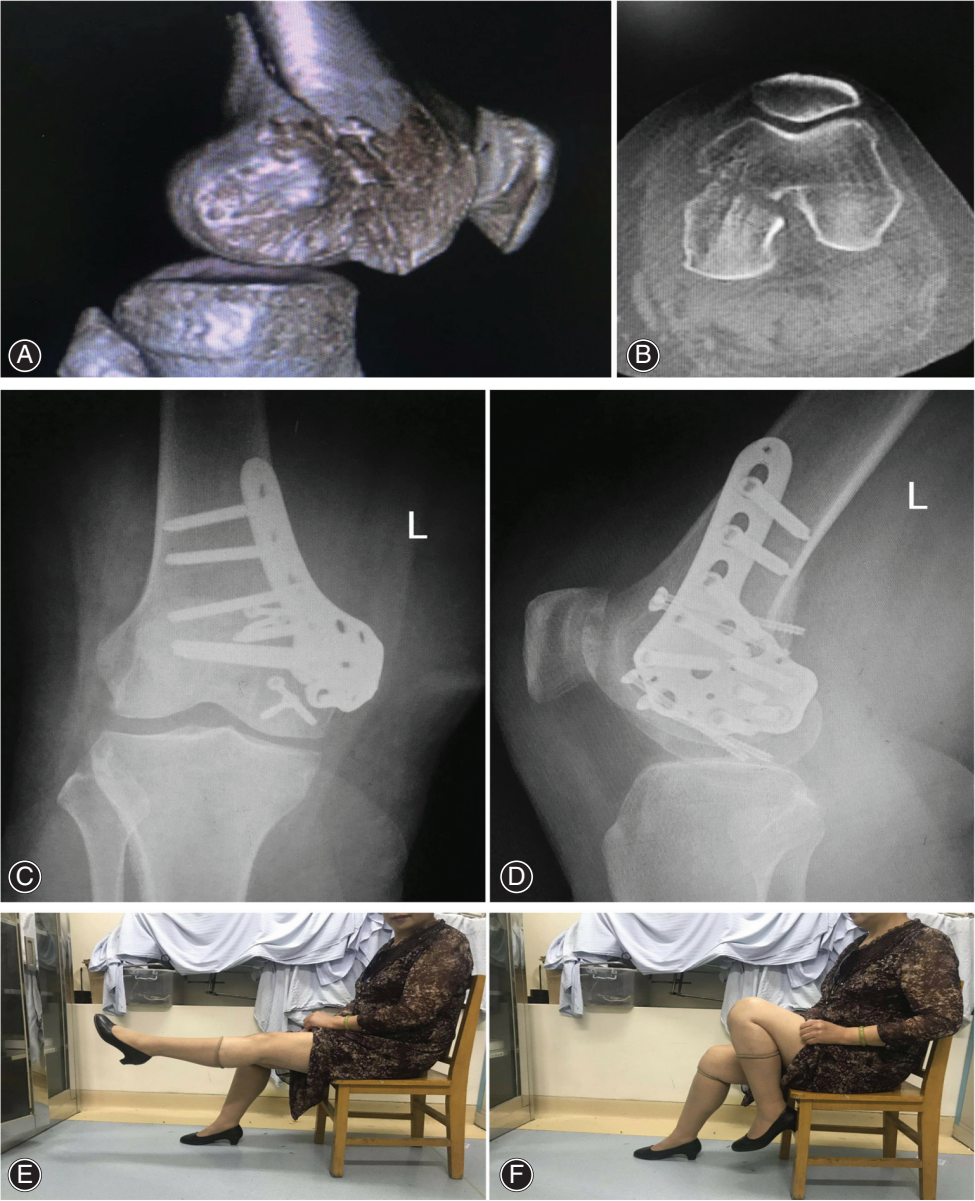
A 60‐year‐old female patient suffered from Hoffa fracture due to a high‐velocity fall. CT of left knee showed a type Letenneur III medial Hoffa fracture (A, B). Radiographs showed that the patient underwent open reduction and internal fixation, and a DFCLP and four cannulated screws were placed at the medial distal femoral condyle (C, D). The patient could acquire nearly 0°–130° range of motion after 24 months postoperatively with a good functional recovery (E, F)
